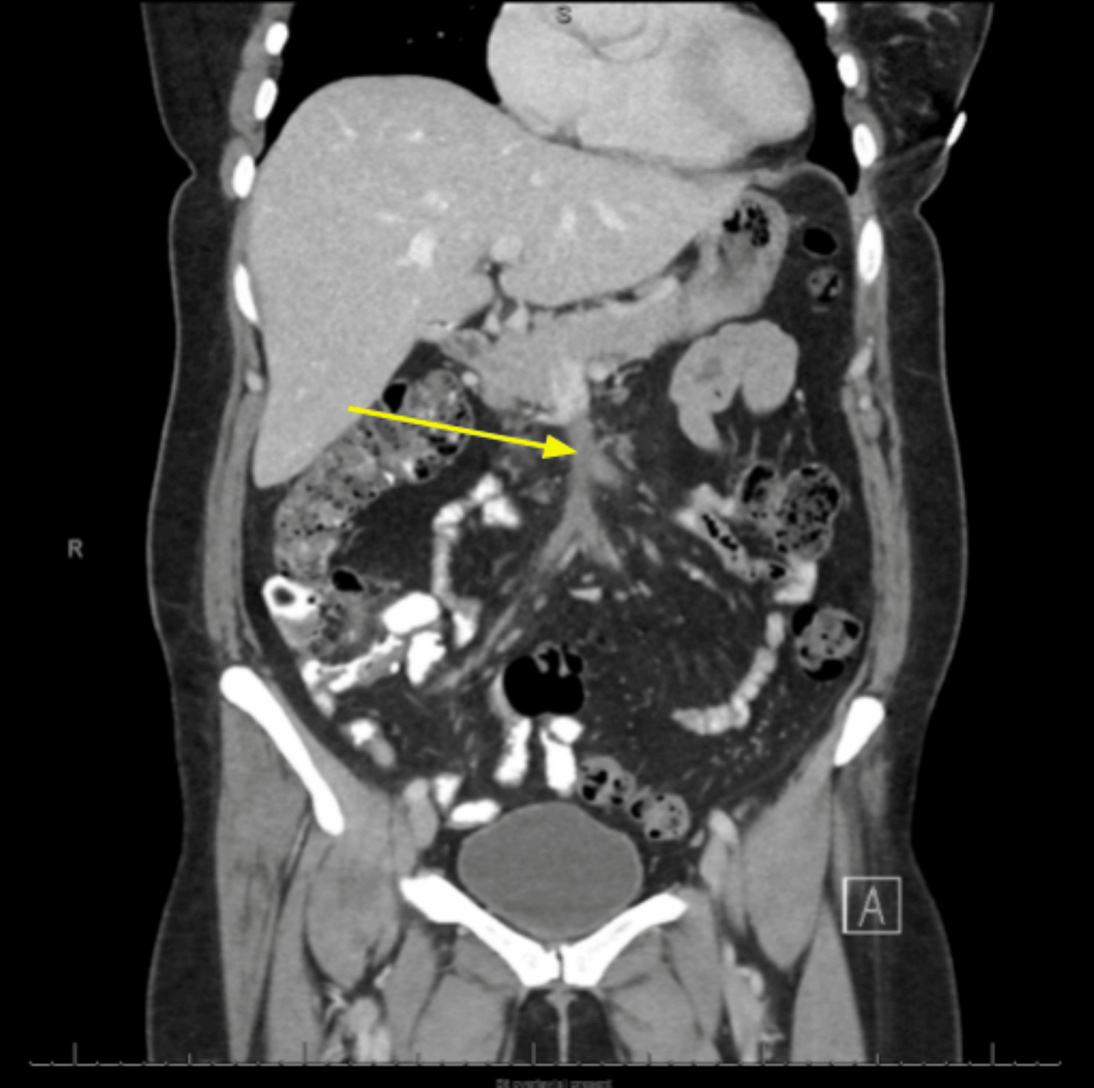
Coronal contrast-enhanced CT angiography of the abdomen revealing a non-opacified filling defect in the superior mesenteric vein (yellow arrow) CT: computed tomography
